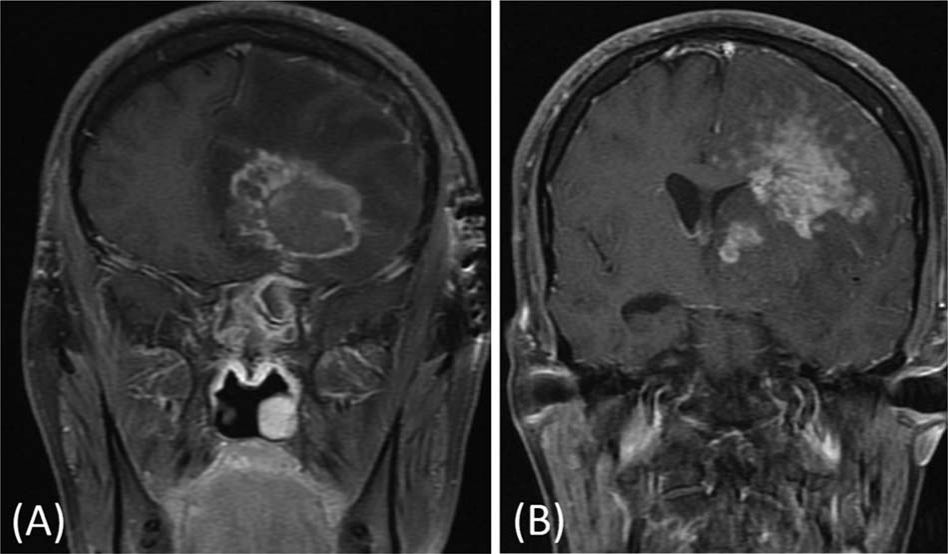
(A and B) Brain MRI with contrast showing signs of intracranial abscess in the left side with mass effect and midline shift to the right side.
